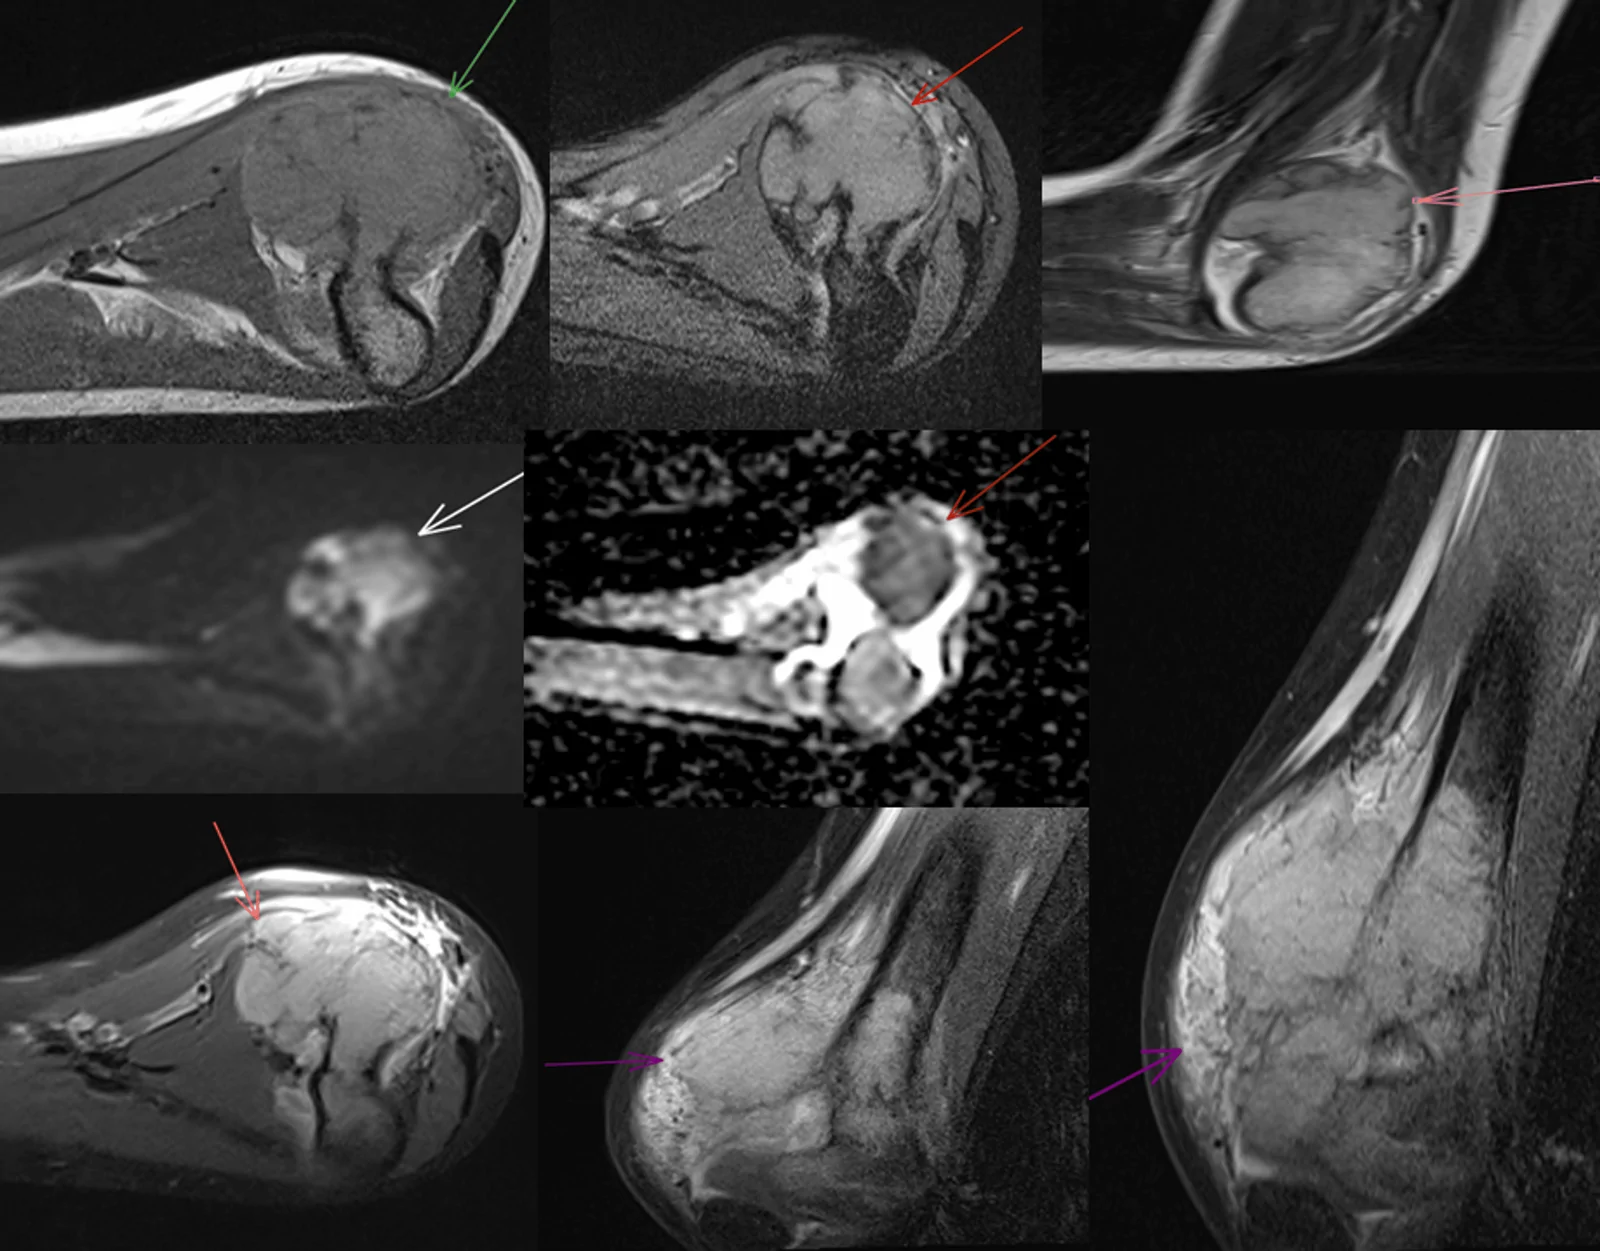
MRI images in axial, coronal, and sagittal planes: showing an aggressive epiphyseal-metaphyseal lesion of the right distal humerus centered on the medial epicondyle, appearing as a mild T1 hyper-intensity (green arrow), an intermediate T2 hyper-intensity (pink and red arrows), and diffusion restriction (white and brown arrows), with enhancement following gadolinium injection (purple and orange arrows).
